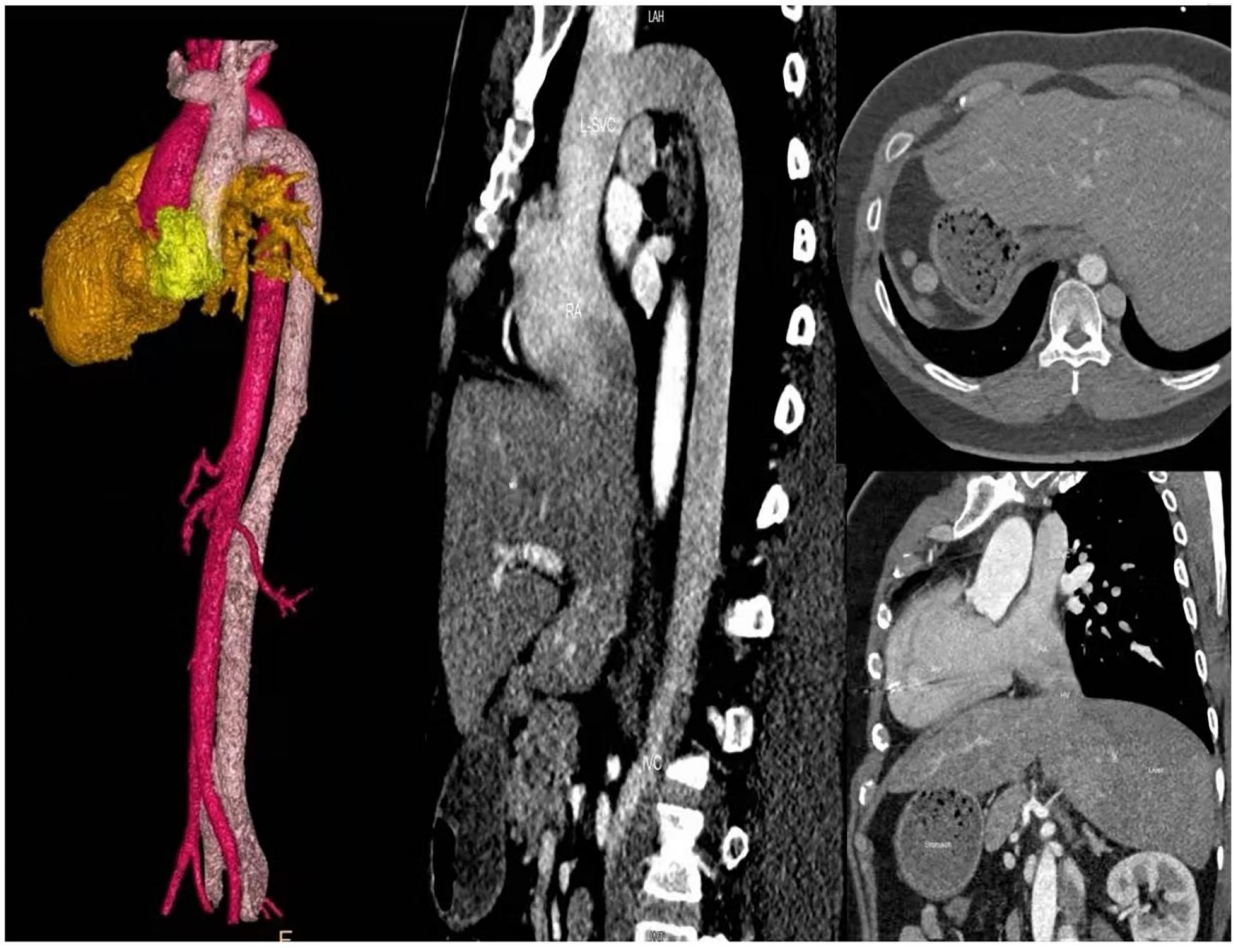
Chest and abdominal large vessel CTA: Absence of the hepatic segment of the inferior vena cava (IVC); the left-sided azygos vein is thickened and extends to the right superior vena cava (SVC); hepatic veins drain directly into the right atrium.
